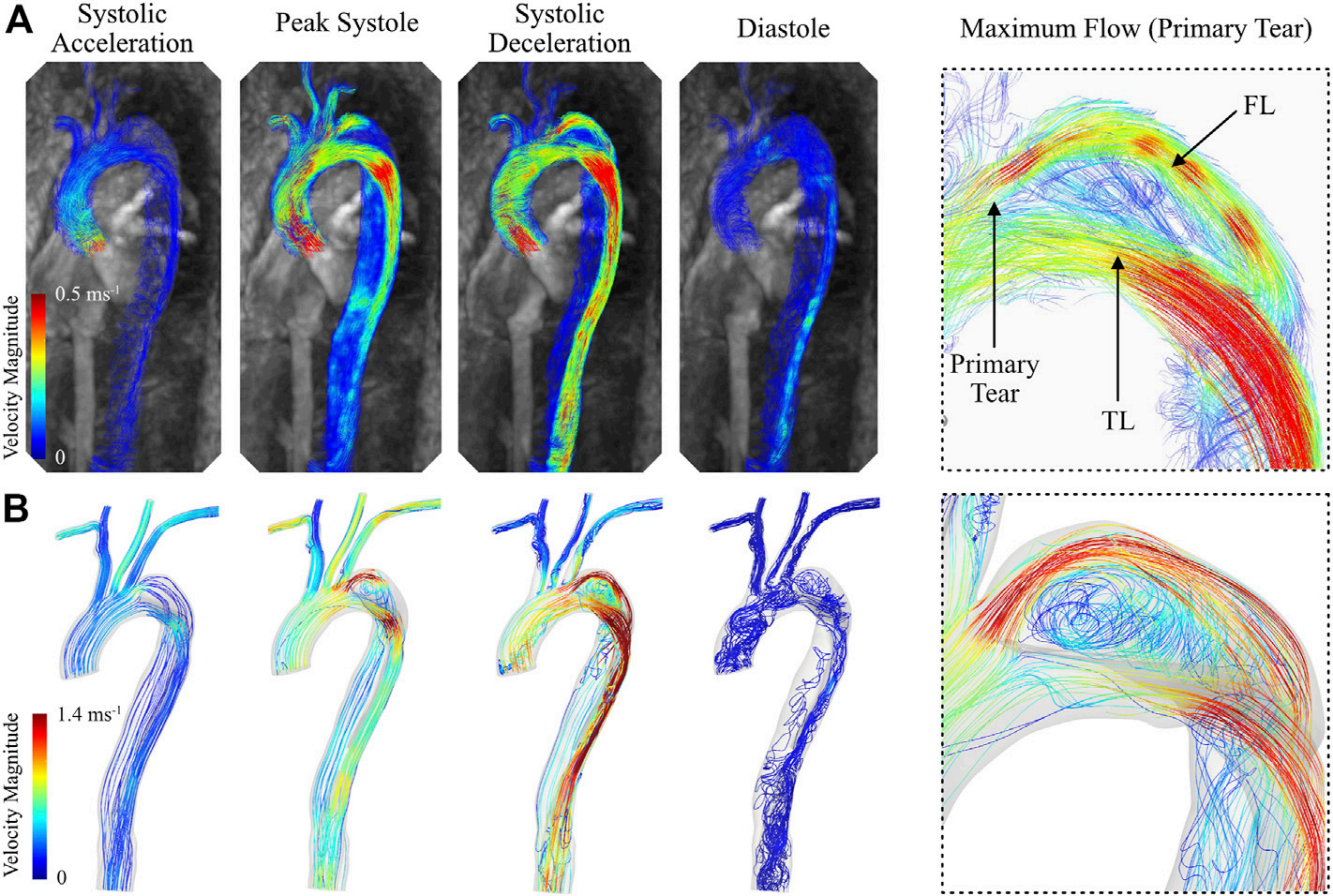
Instantaneous blood velocity magnitude streamlines of the type B thoracic aortic dissection obtained from (A) 4D Flow-MRI and (B) CFD modelling at systolic acceleration, peak systole, systolic deceleration, and diastole. Also visible is the maximum velocity within the FL immediately distal to the primary tear, as extracted from both CFD and 4D Flow-MRI.
